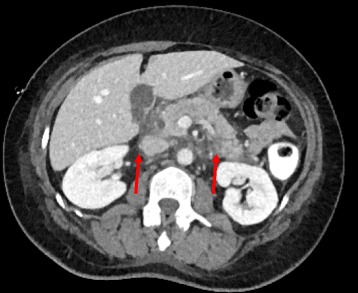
Arterial phase contrast CT showing an acute pancreatitis with edema around the pancreas (*red arrows*)
